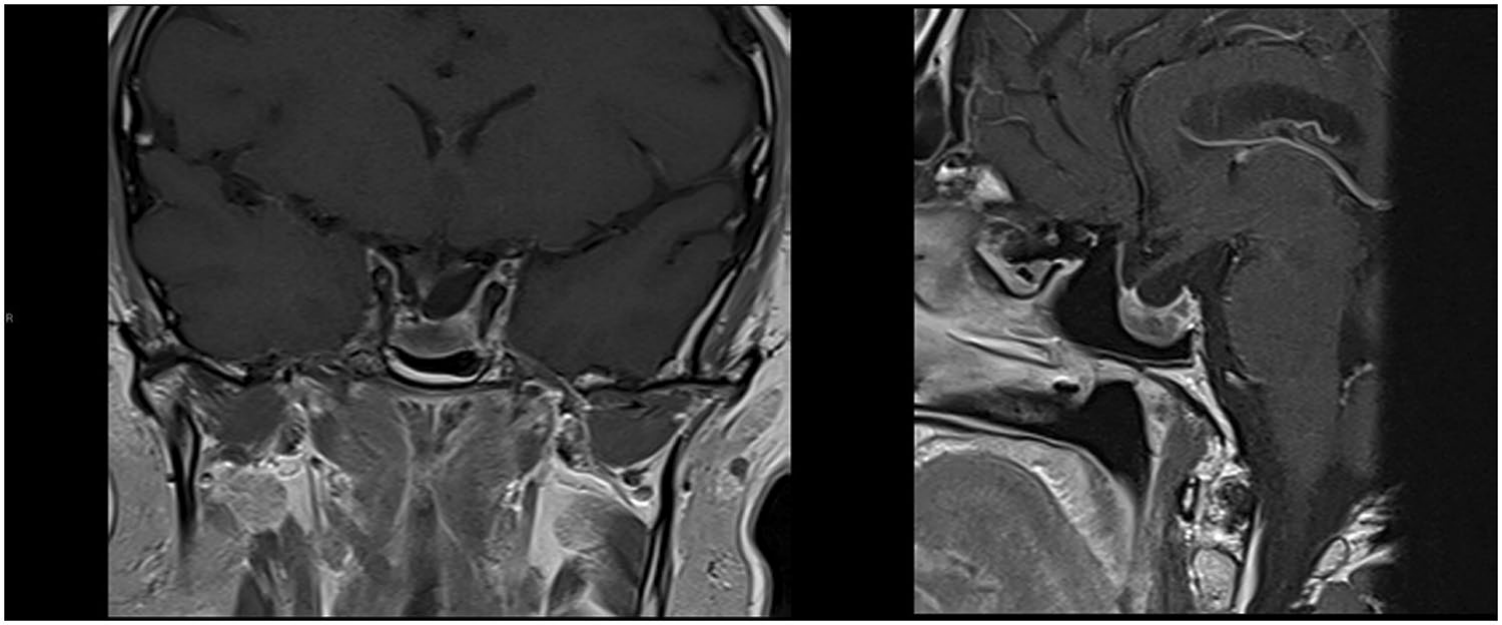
Coronal (left) and sagittal (right) MRI images following treatment
